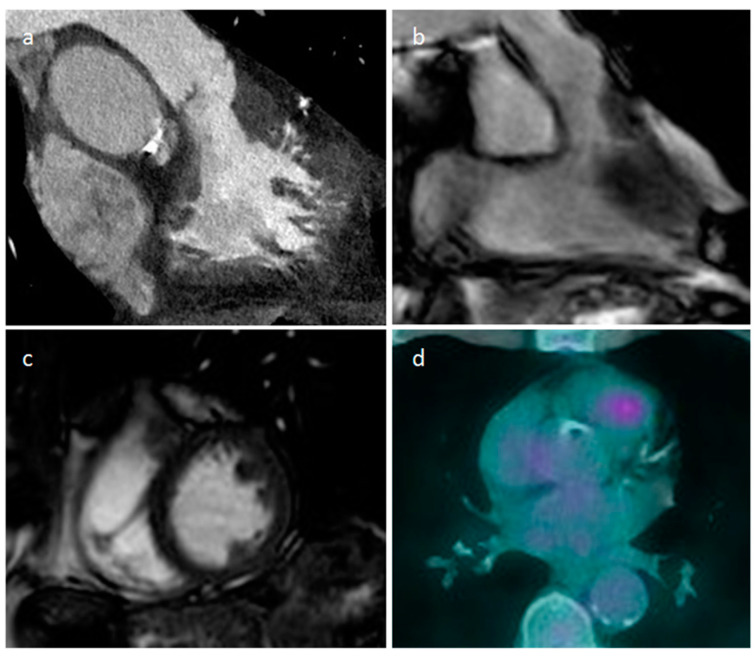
A 72-year-old male patient with right ventricular outflow tract colorectal cancer metastasis in CT scan (a), MR imaging (b,c) and FDG PET/CT scan (d).
